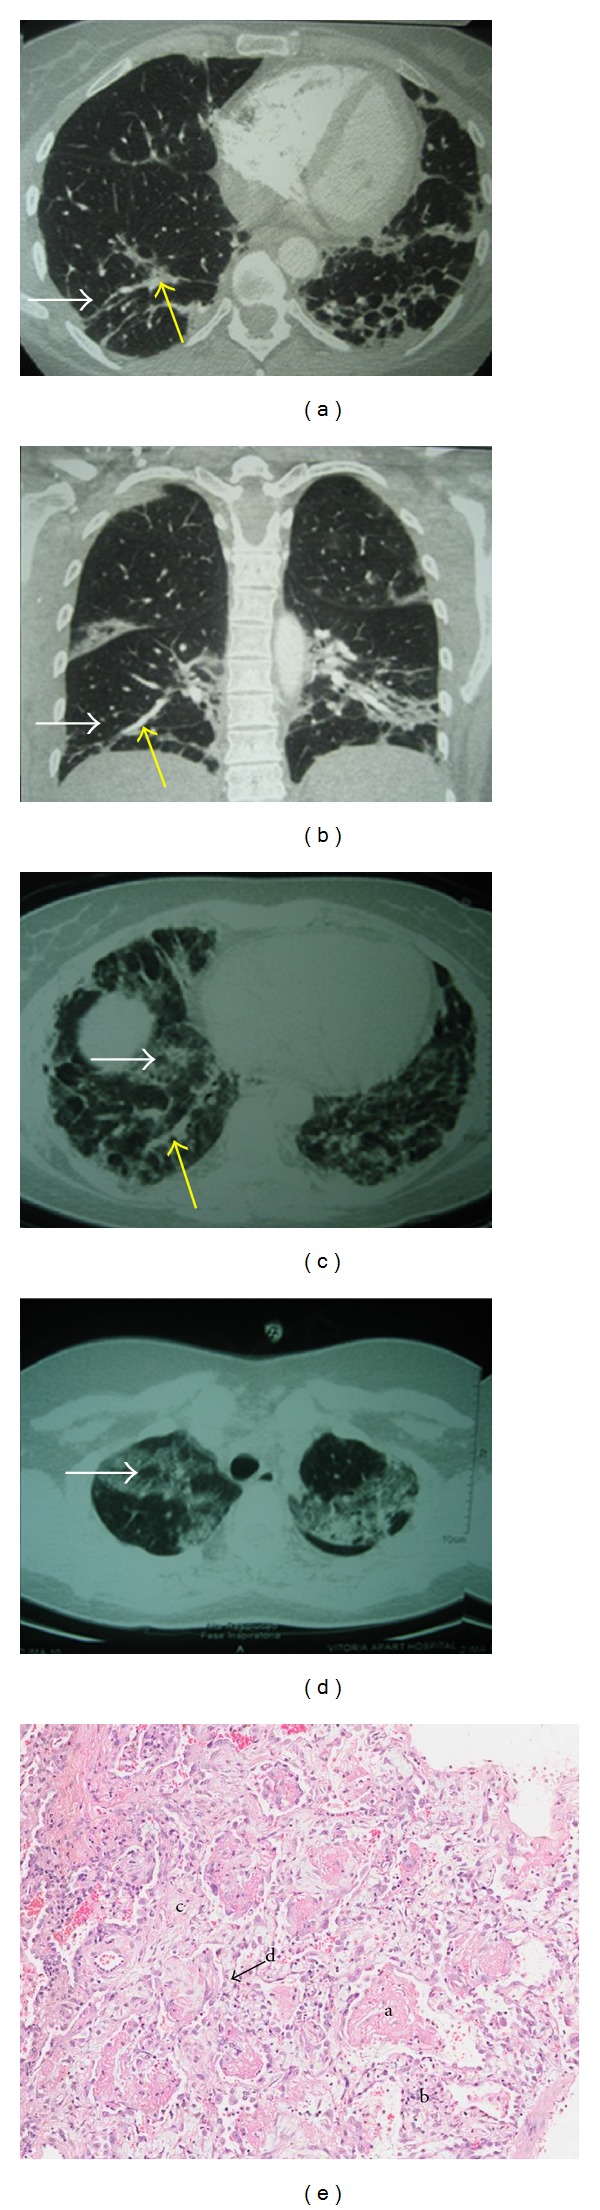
(a) and (b) CT findings of “focal areas of lung parenchymal infiltrates with ground-glass opacities, associated (white arrow) with septal thickening (yellow arrow), with peripheral distribution.” (c) and (d) CT findings of interstitial infiltrate, with diffuse ground glass opacity with foci of parenchymal densification (yellow arrow) and some air bronchograms (white arrow). (e) Histhopatological examination showing (1) intra-alveolar accumulation of fibrin in the form of solid blocks; (2) acute inflammatory infiltrate in the interstitium; (3) alveolar septal edema and congestion; (4) *xantomatososum* macrophages accumulation.
